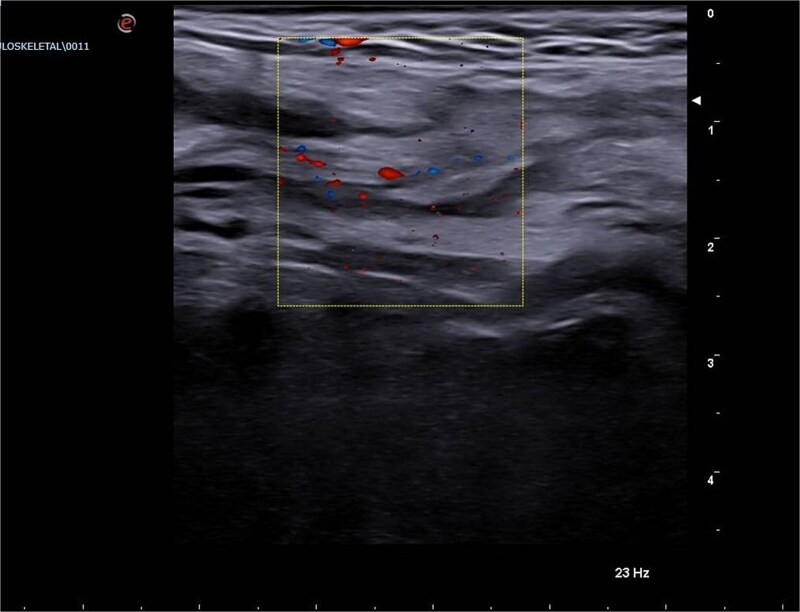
Emergency Department ultrasound images showing a hyperechoic mass occupying the right inguinal canal, with fine vascular signals on color Doppler. The lesion exhibited no change during the Valsalva maneuver and was initially suspected to be an incarcerated omental hernia.
